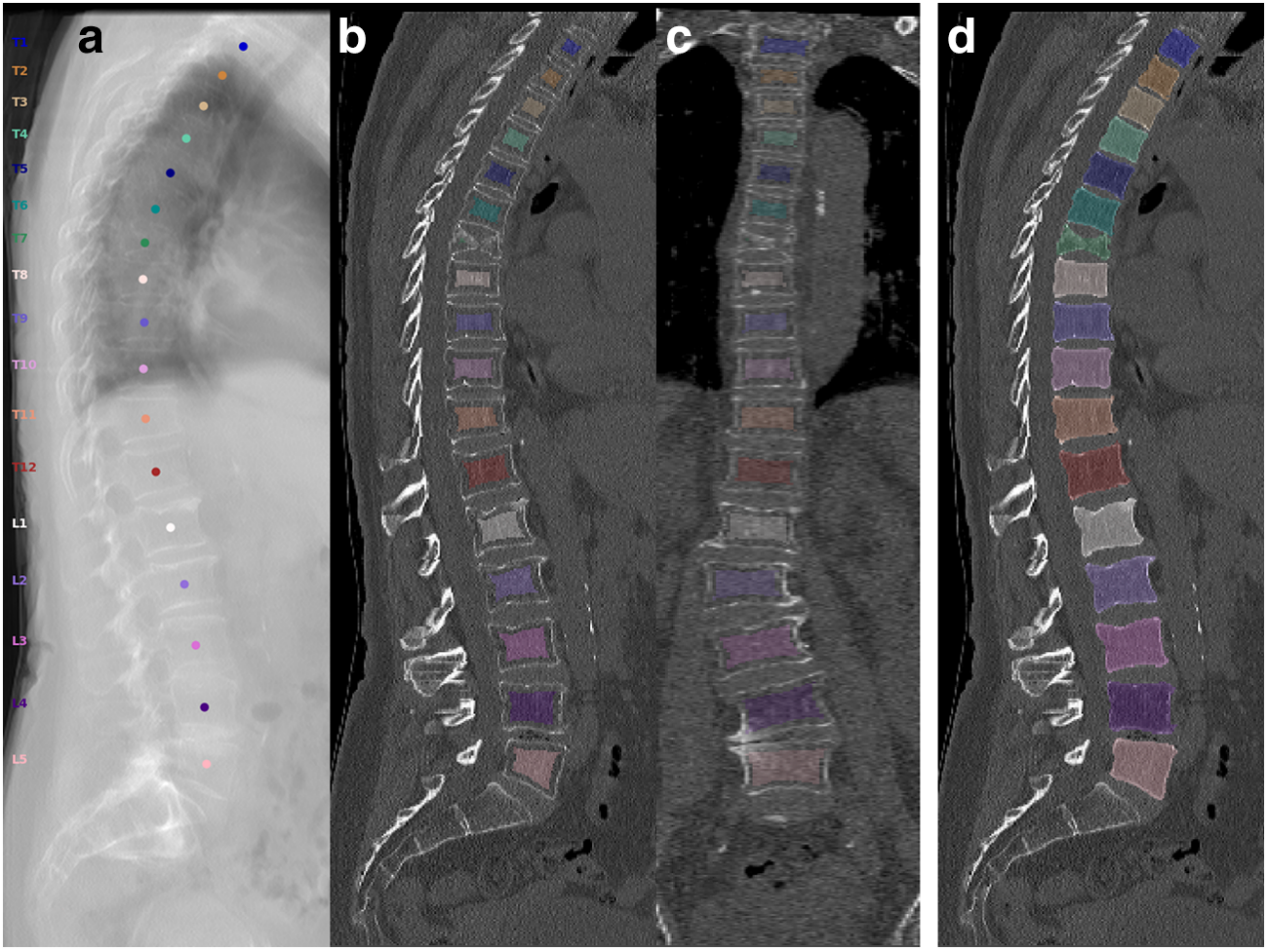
CT scan of an 80-year-old woman with acute back pain visualized as virtual radiograph in lateral projection (a) and curved planar reconstructions in lateral and coronal views (b, c, d). A severe crush fractures is visualized at T7 besides multiple mild to moderate vertebral deformities. Mild scoliotic deformity at the thoracolumbar junction and spondylosis with sclerosis (equivalent to Modic III in MRI) is present at L4/5. Therefore, L4 was excluded from assessment. T12 to L3 yielded a mean trabecular vBMD of 26.6 mg/cm^3^, integral vBMD of 135.4 mg/cm^3^, CT-based aBMD of 0.768 g/cm^2^, and BMC of 4.45 g. Trabecular and integral vBMD are clearly in the osteoporotic range. DXA reported T-score of − 2.5 SD still in the range of low bone mass (not shown). Masks for extraction of trabecular (b, c) as well as integral vBMD (d) are shown as colored overlays. Colored points in the virtual radiograph are automatically estimated by the labelling CNN and represent the vertebral body centroids. Lateral and coronal curved planes are reconstructed by interpolation through these points
